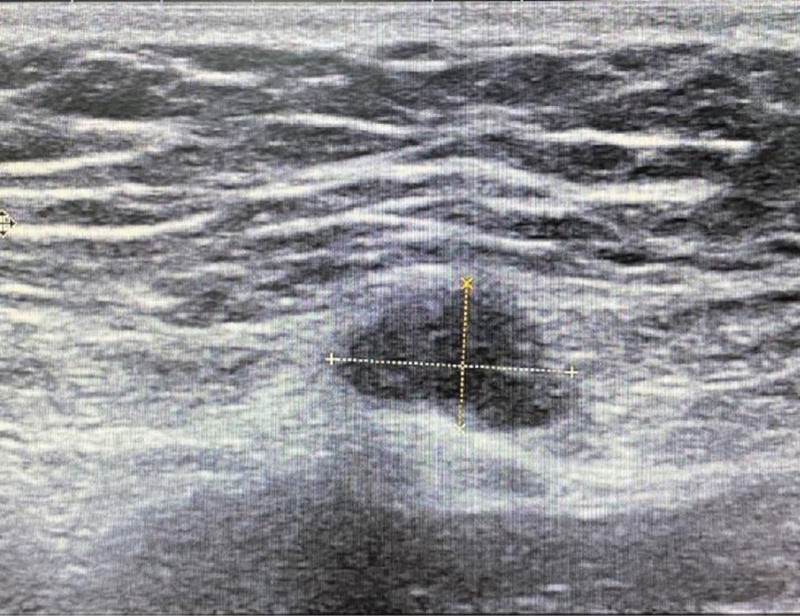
Ultrasound of abdominal wall masses showed a low echo mass in the lower left quadrant area where the surgery scar lied. Its size was about 33 mm × 7 mm × 14 mm, 17.5 mm away from the surface of the abdomen with uneven internal echoes, and the margin was clear.
